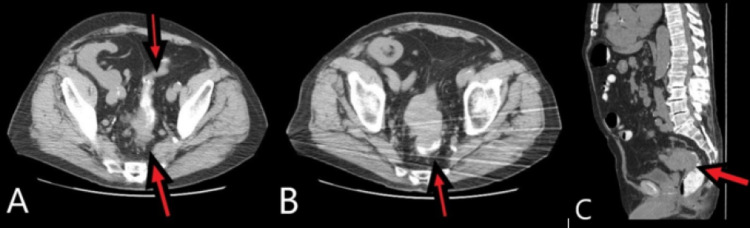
Rectosigmoid malignancy with extension into the peritoneum. (A) Preoperative CT scan, axial view of an 8.8 cm mass at the rectosigmoid junction (red arrow). (B) Preoperative CT scan, axial view of an 8.8 cm mass at the rectosigmoid junction (red arrow). (C) Preoperative CT scan, sagittal view of locoregional spread and lymphovascular invasion (red arrow). CT: computerized tomography
